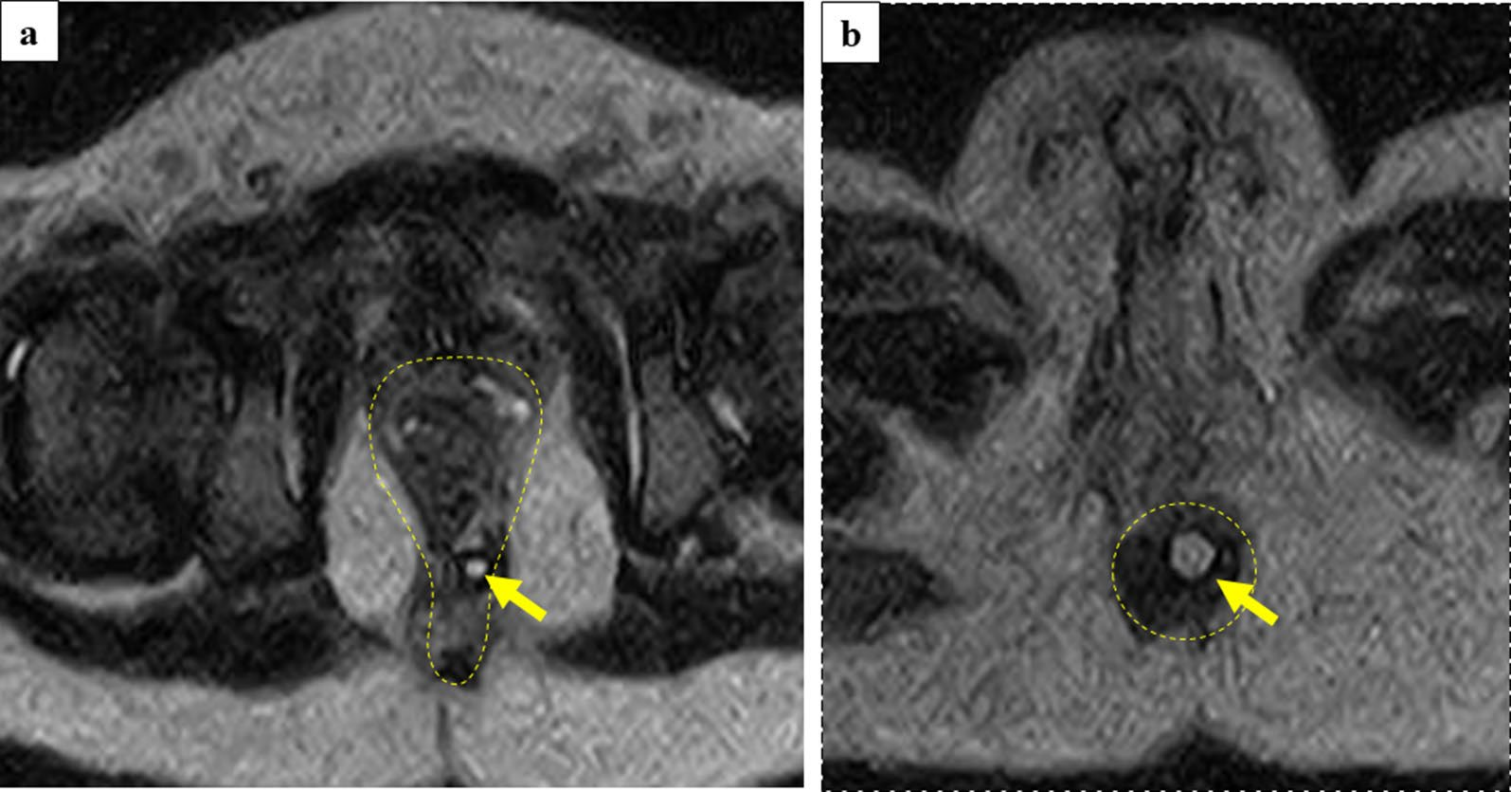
T2-weighted images (axial view). a A 0.45-T open MRI scan clearly revealed the rectum in the center of muscle complex (outlined by dot line). To clarify rectal pull-through, a 1 ml syringe filled with physiological saline was inserted in the anus (arrow). b Dot line showed the external sphincter muscle. Pull through rectum (1 ml syringe filled with physiological saline: arrow) was confirmed at the center of the external sphincter muscle
